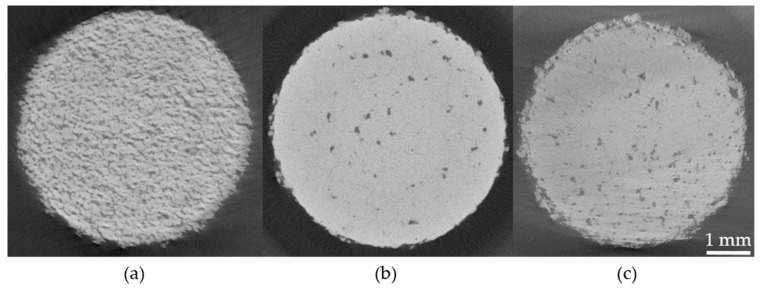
X-ray computer tomograms of (a) pure copper cylinder, (b) Coated Sn3.0 and (c) Coated Ni1.9. The cracks in the samples made from coated powder are visible and no loose powder is found in the pores. All tomograms are from samples produced at 100 mm/s scanning speed.
